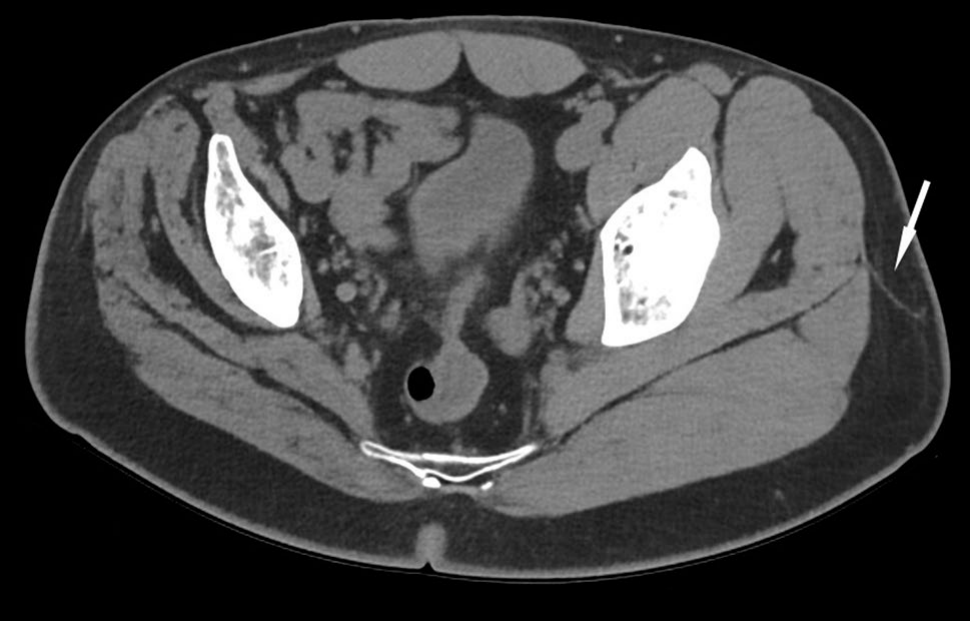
Perforating vessel (white arrow) that perforates the anterior border of the gluteus maximus, in an axial CT scan of the pelvis
